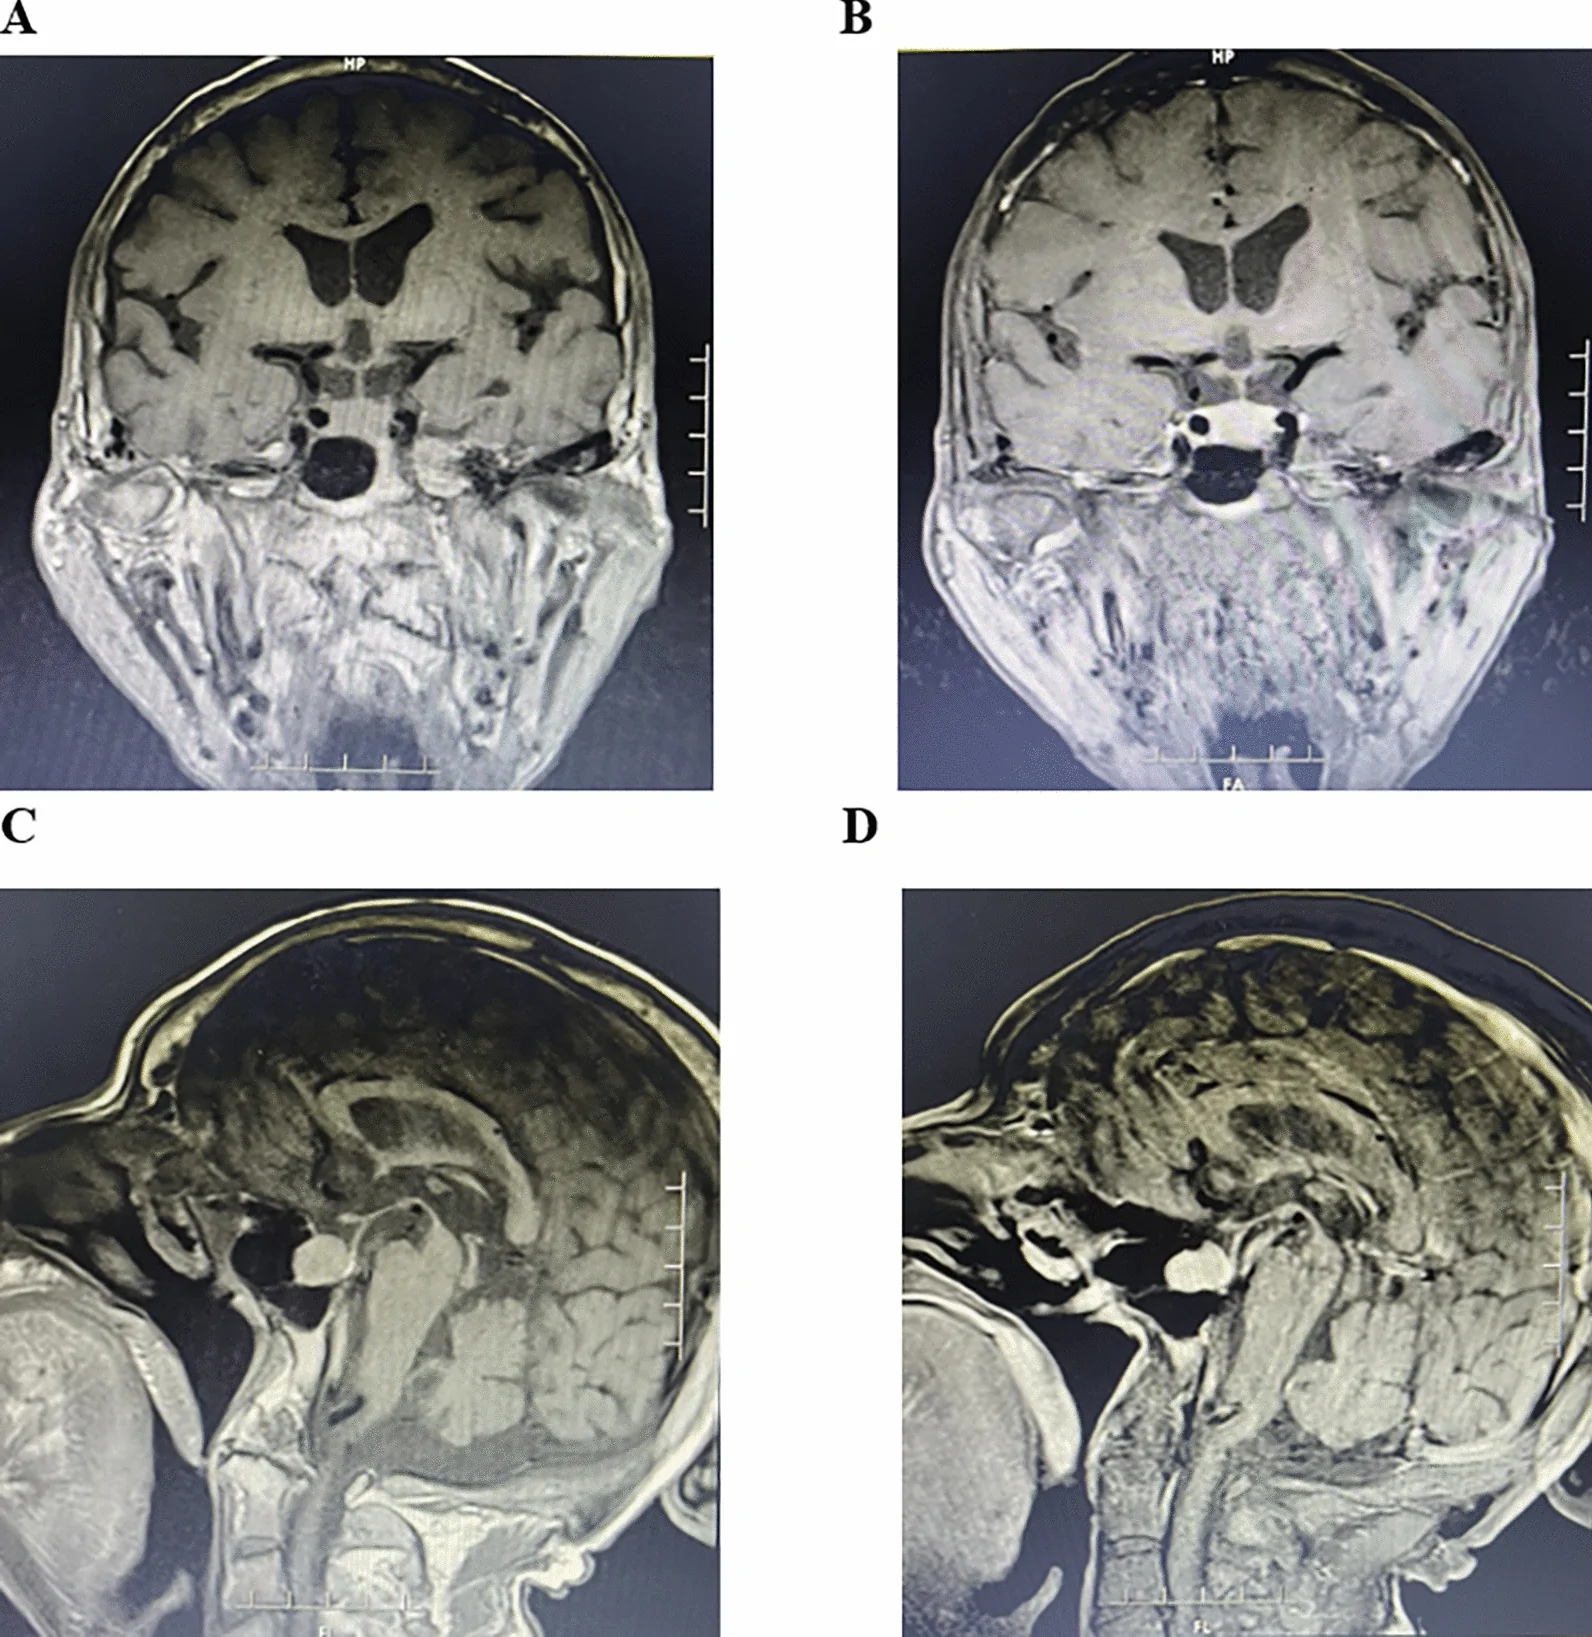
A Precontrast T1-weighted coronal slices magnetic resonance imaging (MRI) image. B Postcontrast T1-weighted coronal slices MRI image. C Precontrast T1-weighted sagittal slices MRI image. D Postcontrast T1-weighted sagittal slices MRI image
